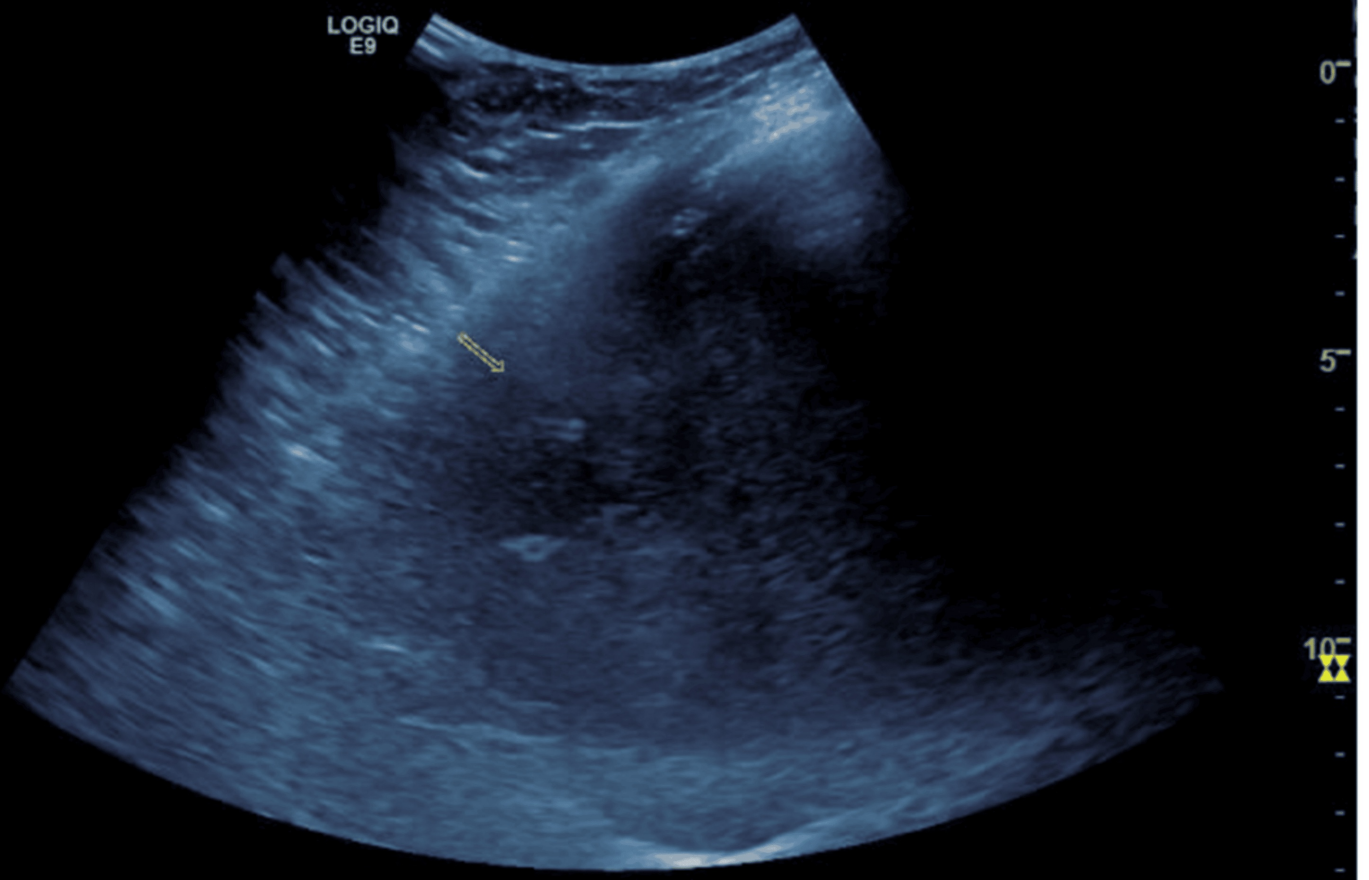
This ultrasound image depicts the right upper quadrant of the abdomen, revealing a hypoechoic abscess in the right hepatic lobe. The abscess measures approximately 4.4 cm x 3.1 cm x 4.0 cm and contains a partially imaged pigtail drainage catheter. The central components of the abscess exhibit liquefaction. The yellow small arrow indicates the right hypoechoic abscess.
